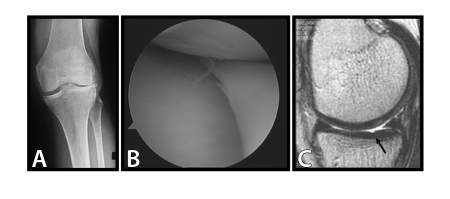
Images of a joint using (a) x-ray (b) arthroscopy and (c) MRI.
